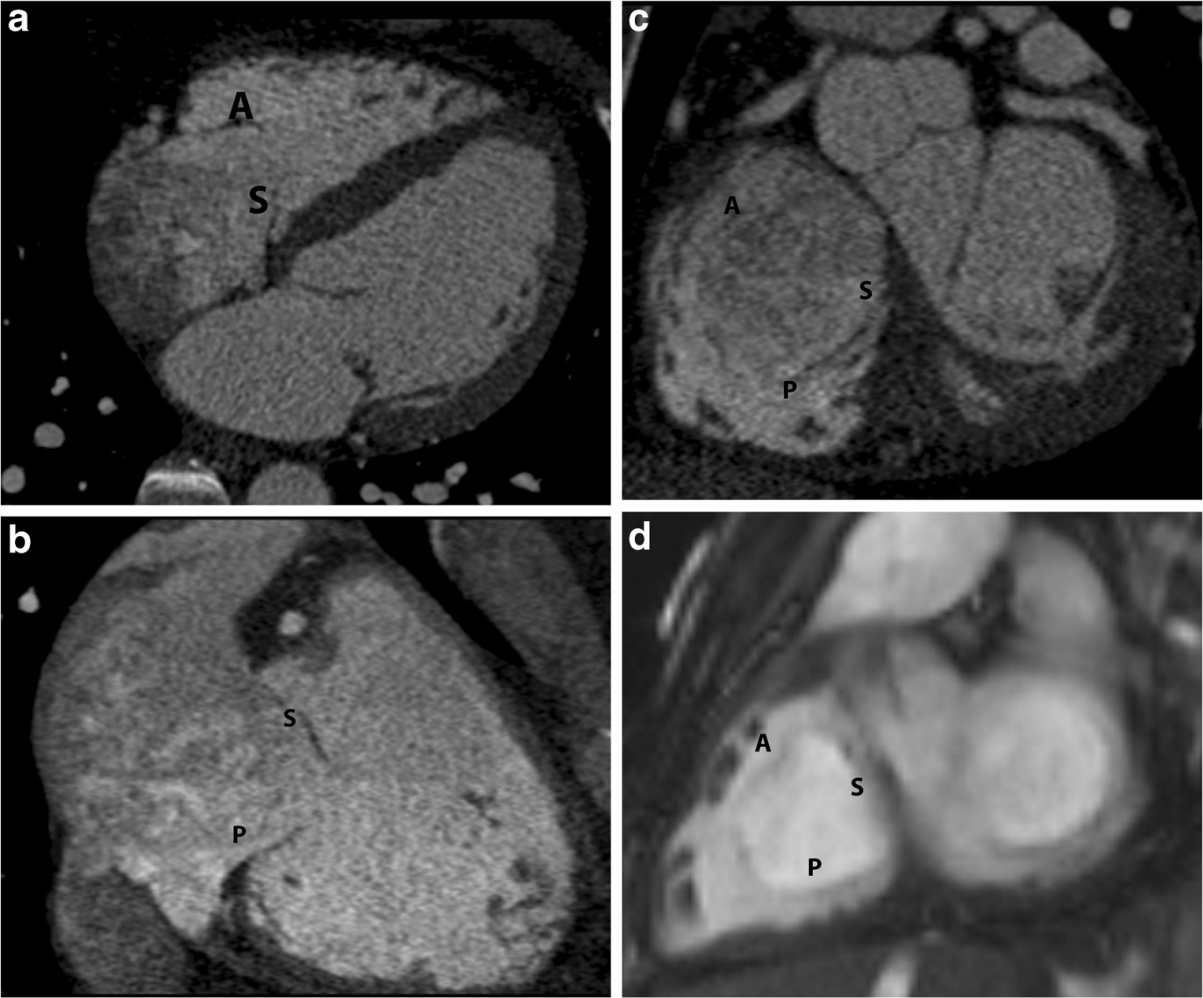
Normal appearance of tricuspid valve. (a) Four-chamber CT image shows the septal (S) and anterior (A) leaflets. (b) Two-chamber CT image shows septal (S) and posterior (P) leaflets. (c) Short-axis CT image shows all the leaflets (S, A, P). (d) MRI appearance of the tricuspid annulus, with the short-axis steady-state free precession (SSFP) image showing all the leaflets (S, A, P)
